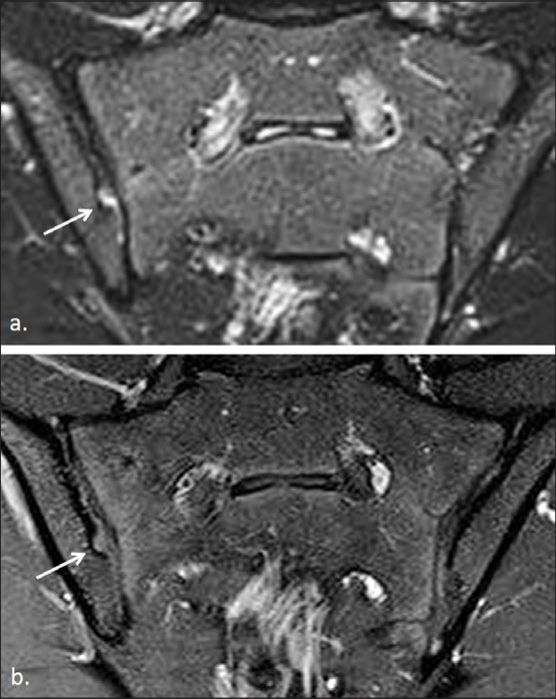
Active sacroiliitis in a 15-year-old boy with JSpA according to a global assessment of MRI for sacroiliitis, not according to the ASAS definition of a positive MRI for sacroiliitis. a. Semicoronal STIR image shows a focal nodular high signal in the right sacroiliac joint representing an active erosion (arrow). b. Semicoronal contrast-enhanced fat-saturated T1-weighted image shows corresponding enhancement of this lesion (arrow).
